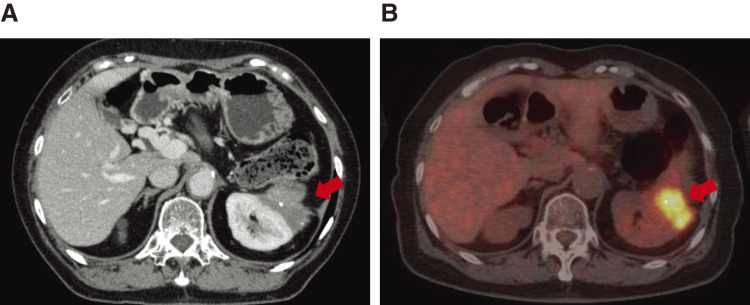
Radiological examinations of dissemination. (A) CT showing soft tissue in front of left kidney (arrow). (B) Positron emission tomography (PET)/CT shows fluorodeoxyglucose (FDG) uptake in the tissue (arrow).
